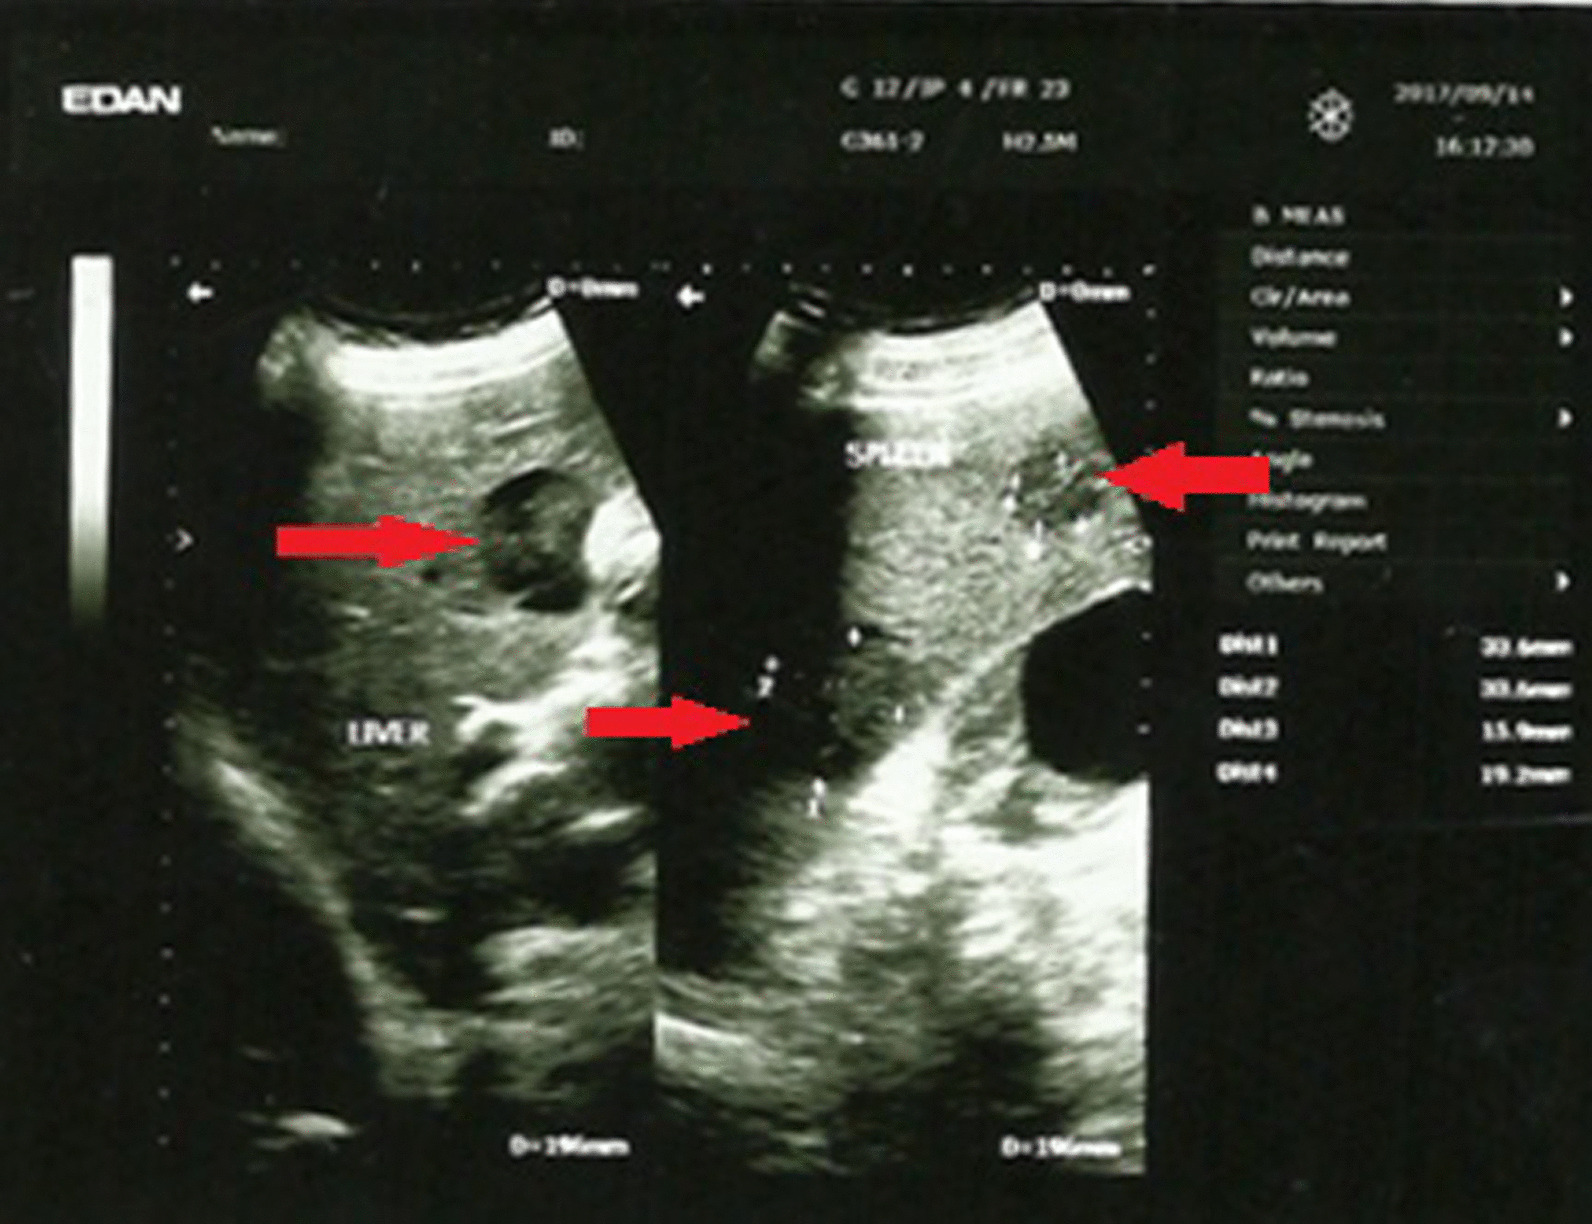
Ultrasound of the abdomen, showing a node in the liver (arrow, left) and intrasplenic nodes (arrows, right)
